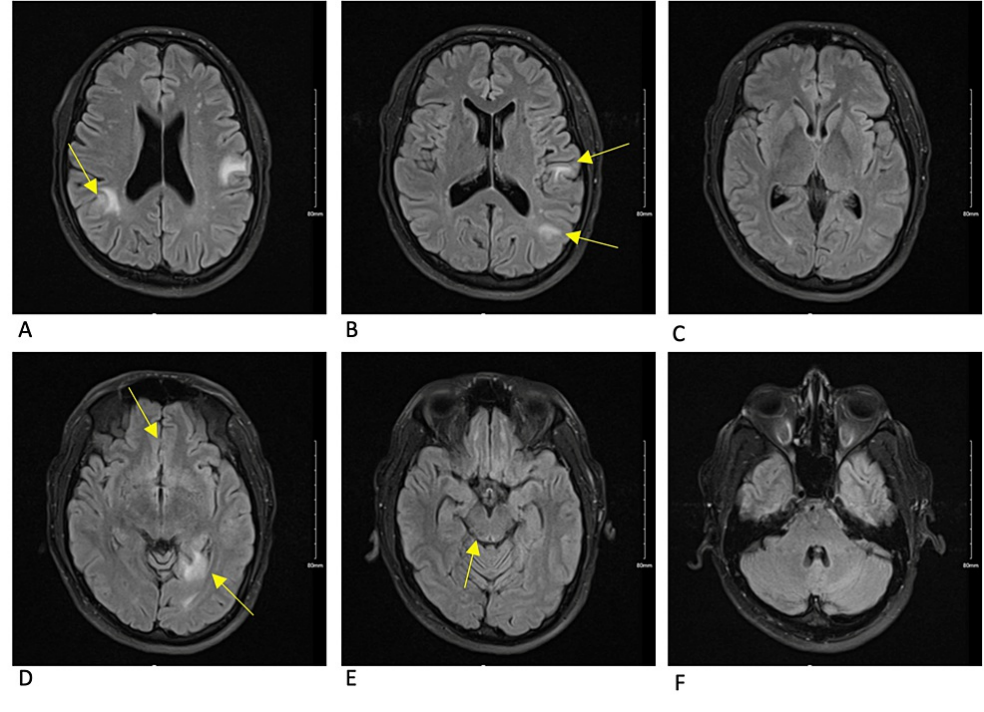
MRI brain T2 flair sequence five days after initiation of dual antiparasitic therapy An interval decrease in the size of the ring-enhancing lesions consistent with early response to antiparasitic treatment is demonstrated. The inflammation associated with the anterior right and left frontal lobes is resolved (panes A and B). The cysts in the left and right parietal lobes have decreased perilesional edema (panes A and B). The interhemispheric cyst and right posterior midbrain cyst have decreased in size (panes D and E). The inflammation associated with the hippocampal cyst has minimally increased (pane D).
